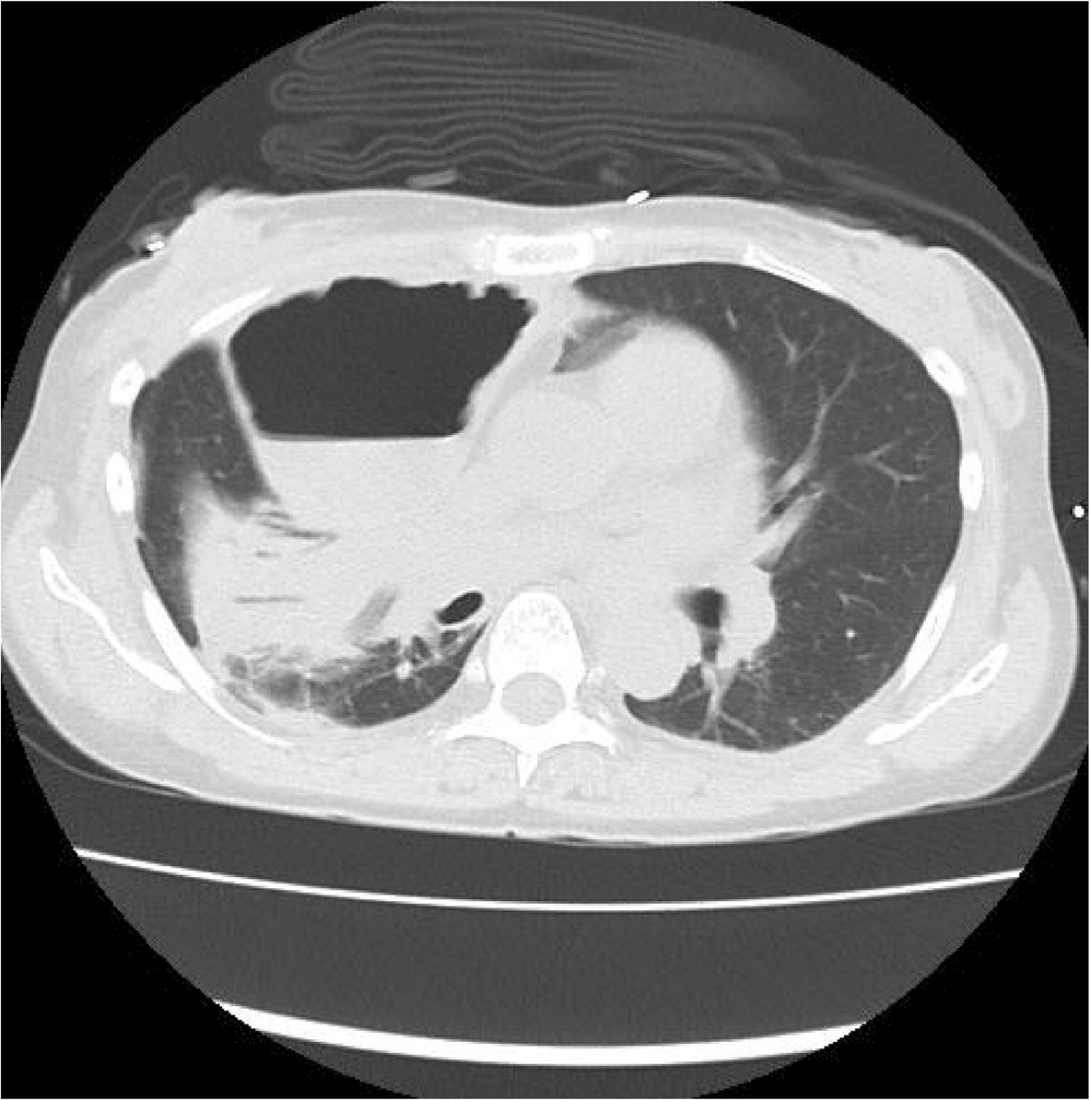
Chest computed tomographic image showing transverse section of the distended, reconstructed whole stomach compressing the proximal lung parenchyma
